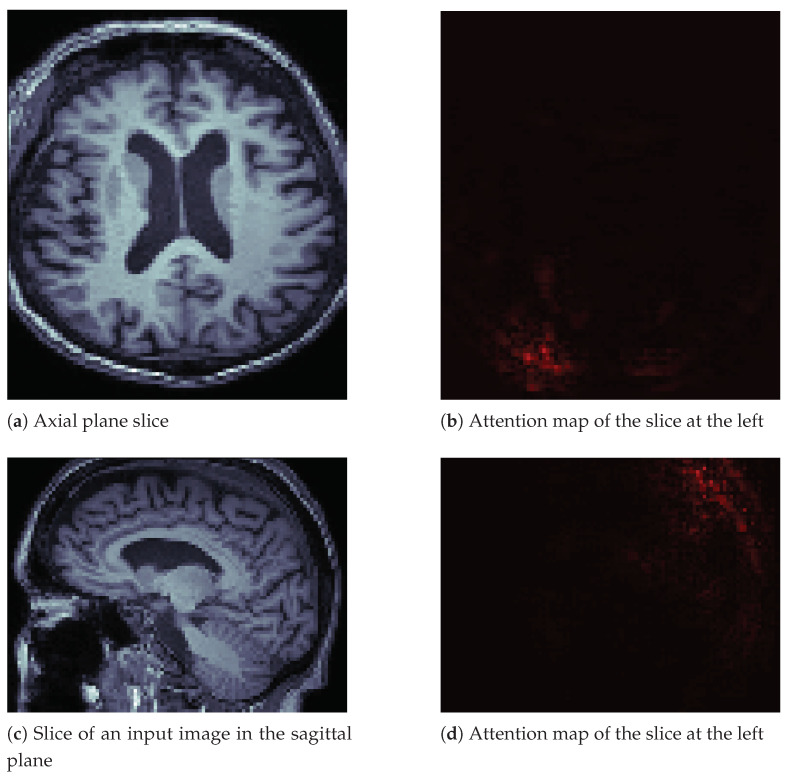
Image slices and their attention maps from an MRI of a healthy person.
